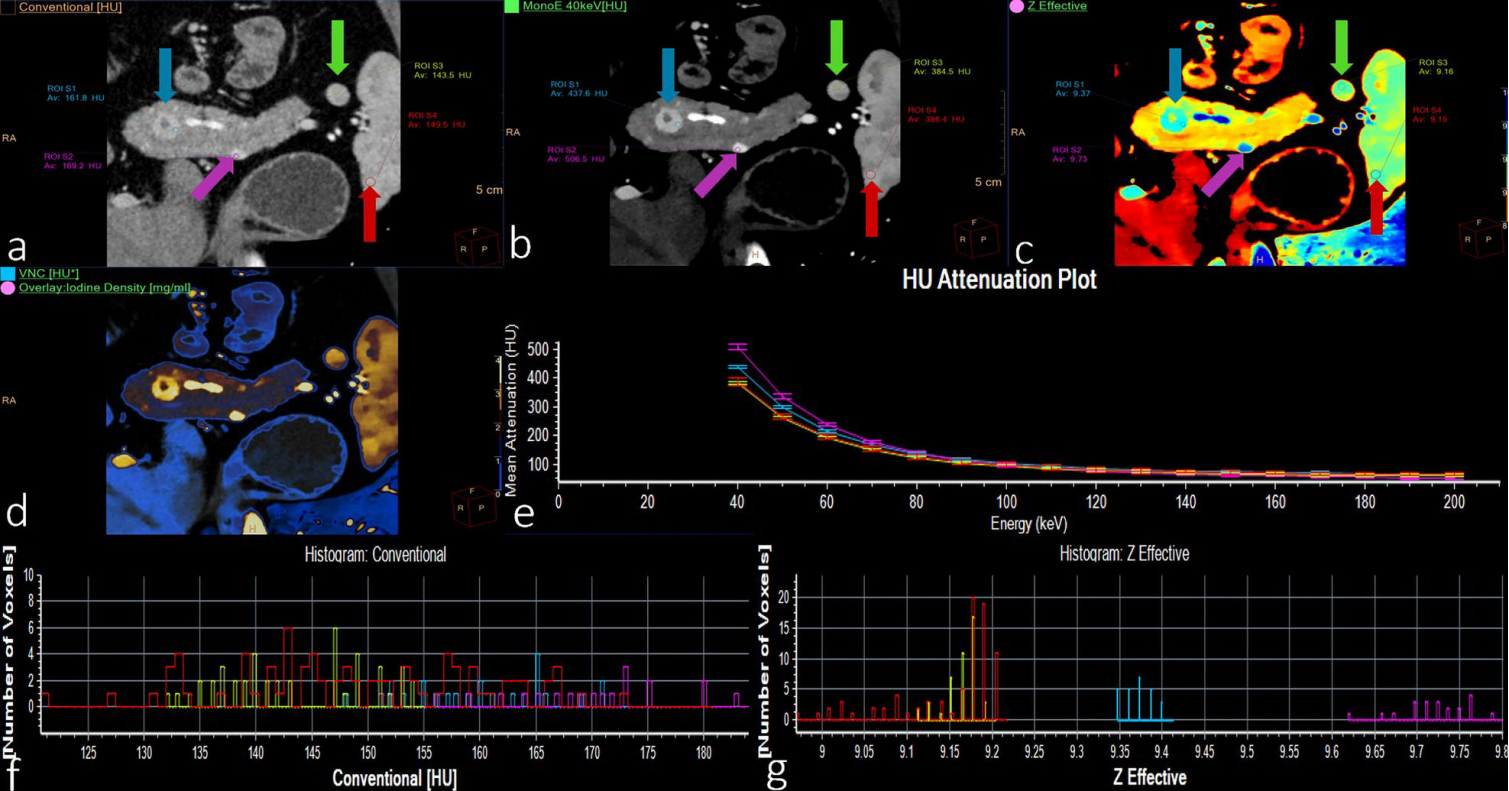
A 34-year-old male patient presented with repeated fatigue for 3 months. The pathological diagnosis was multiple islet cell tumors. There are four regions of interest, including the S1 (tumor, blue arrow), S2 (tumor, purple arrow), S3 (accessory spleen, green arrow), and S4 (spleen, red arrow) lesions. Arterial phase CT and postprocessing images: a conventional images; b 40 keV images; c
